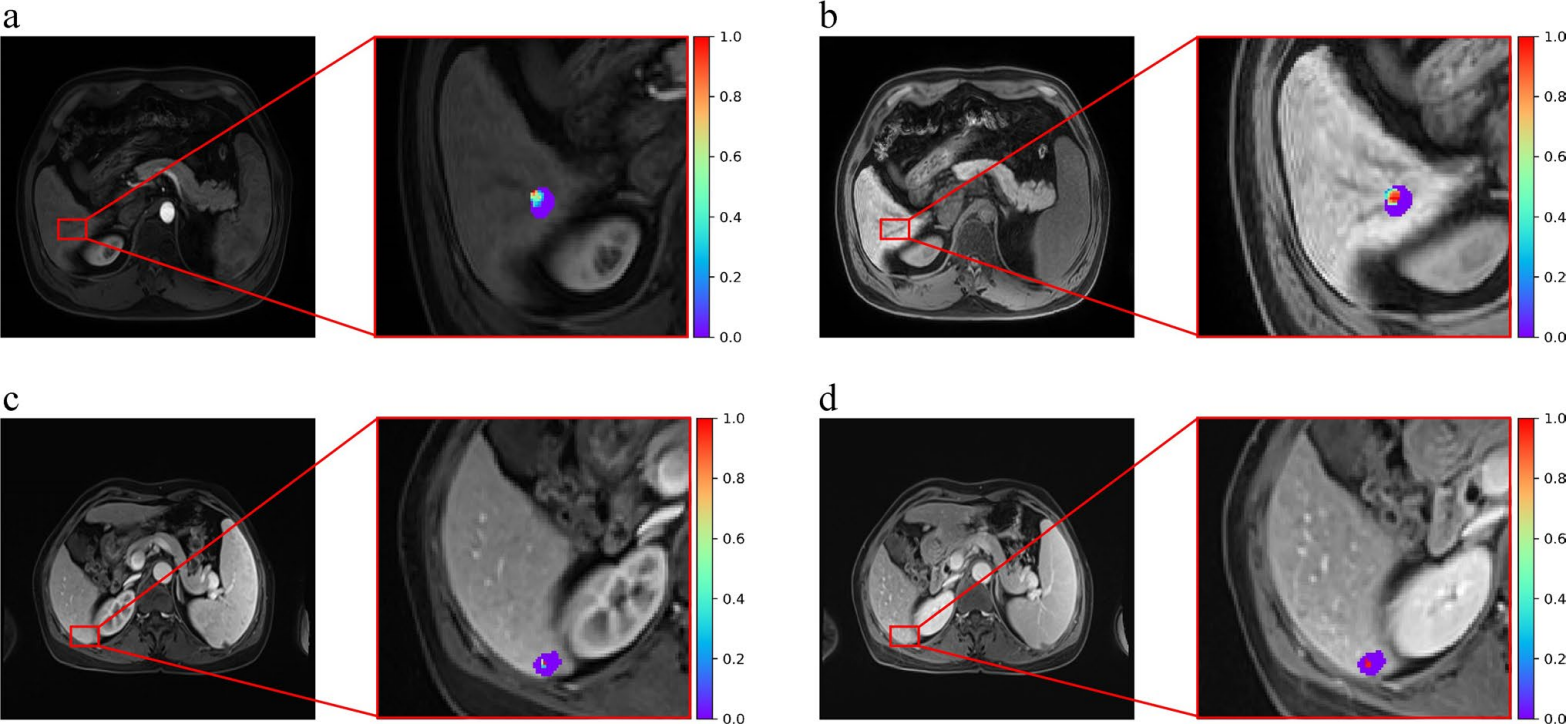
Feature visualization of the gray level dependence matrix (GLDM) of arterial phase MRI by wavelet filter (a). Feature visualization of the first order Range of T1WI phase MRI by Laplacian of Gaussian (LoG; *σ* = 2 mm) filter (b). Feature visualization of the gray level size zone matrix (GLSZM) of arterial phase MRI by wavelet filter (c). Feature visualization of the first order Maximum of delayed phase MRI by none filter (d)
